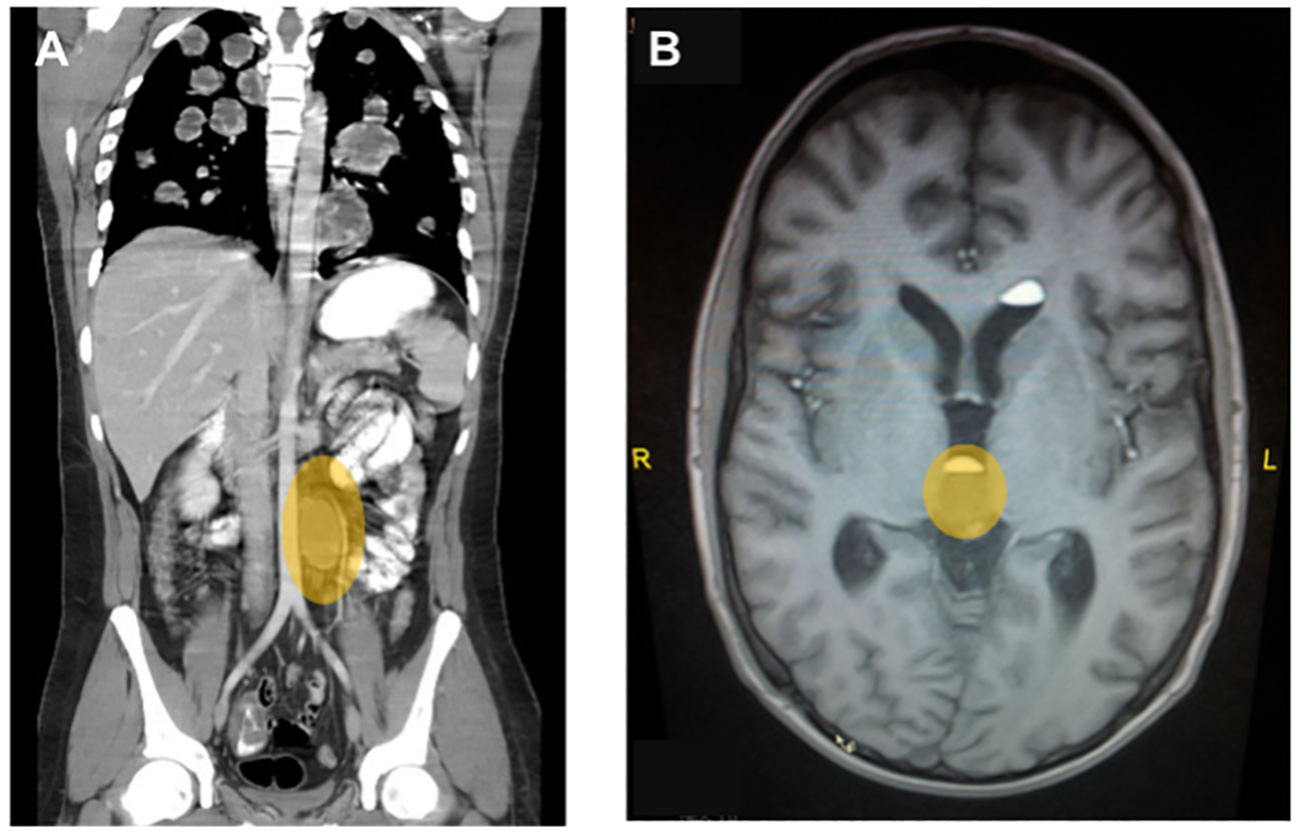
Representative diagnostic images at primary diagnosis for case 2. (A) Contrast-enhanced computed tomography of chest abdomen and pelvis showing multiple bilateral, centrally necrotic lung metastases and a singular retroperitoneal para-aortic lymph node metastasis. (B) T2-weighted image of a whole-brain MRI showing a central metastasis close to the pituitary gland, which lead to a clinically significant diabetes mellitus with polyuria and polydipsia.
